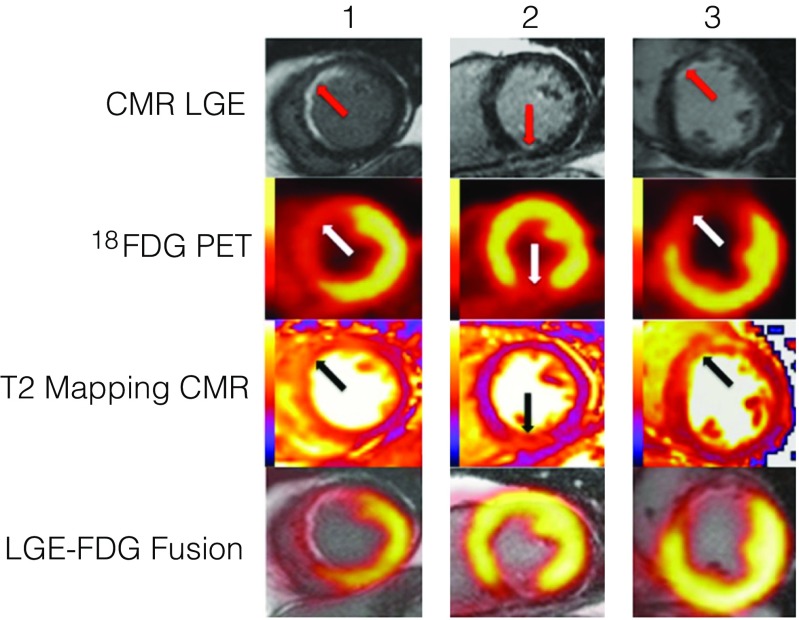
Hybrid positron emission tomography– magnetic resonance (PET-CMR) cardiac imaging of subendocardial infarction. Three study patients with subendocardial myocardial infarction (red arrows) with significant myocardial salvage. The areas of reduced ^18^F–fluorodeoxyglucose (FDG) uptake (white arrows) and the areas of edema on T2 maps (the AAR; black arrows) extended both transmurally and radially beyond the areas of late gadolinium enhancement (LGE). AAR indicates area at risk. Figure from adapted from Bullock et al. *[36]Circ CV Imaging 2016*
